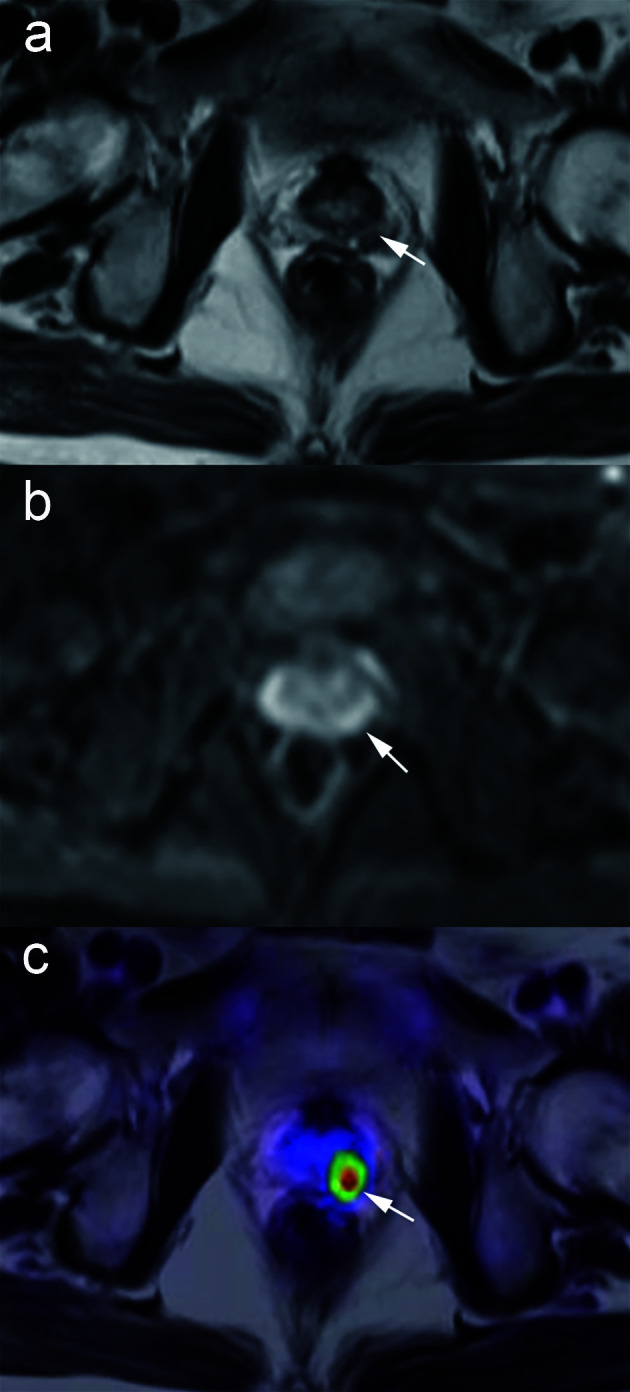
A patient treated with management change in suspected PCa. Images from a 59-year-old male with a PSA level of 7.07 ng/ml. No tumor detection within the prostate is achievable with T2-weighted or DWI sequence alone (A, B), but fused PET/MRI demonstrates tumor involvement of the left lobe (C, white arrow). The management plan was shifted from active surveillance to biopsy. The subsequent prostate biopsy confirmed Gleason 4 + 3 prostate cancer in the ipsilateral lobe.
